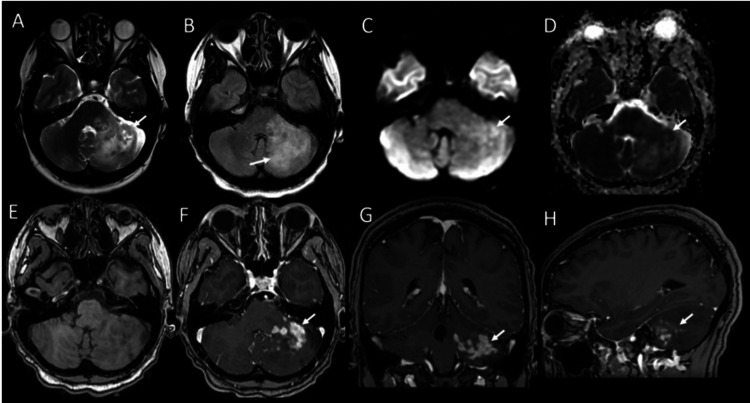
Contrast-enhanced MRI brain Axial T2 (A), FLAIR (B), DWI (C), ADC (D), pre-contrast 3DT1 (E), and postcontrast 3DT1 in axial (F), coronal (G) and sagittal (H) planes demonstrate multiple punctate, patchy, nodular, and linear lesions mainly involving the left middle cerebellar peduncle extending to left cerebellum and left aspect of the pons. Nodular T2 hypointense areas (arrow in A) with DWI hyperintensity (arrow in C) and corresponding ADC hypointensity (arrow in D) representing restricted diffusion compatible with a hypercellular process. There is surrounding mild edema in the left cerebellum and middle cerebellar peduncle on FLAIR (arrow in B); however, without significant mass effect. These lesions demonstrate prominent patchy, nodular, and punctate enhancement after administration of contrast (arrows in F, G, H) suggesting a lymphocytic perivascular inflammatory pattern. ADC: Apparent Diffusion Coefficient; DWI: Diffusion-Weighted Imaging; FLAIR: Fluid Attenuated Inversion Recovery; MRI: Magnetic Resonance Imaging
